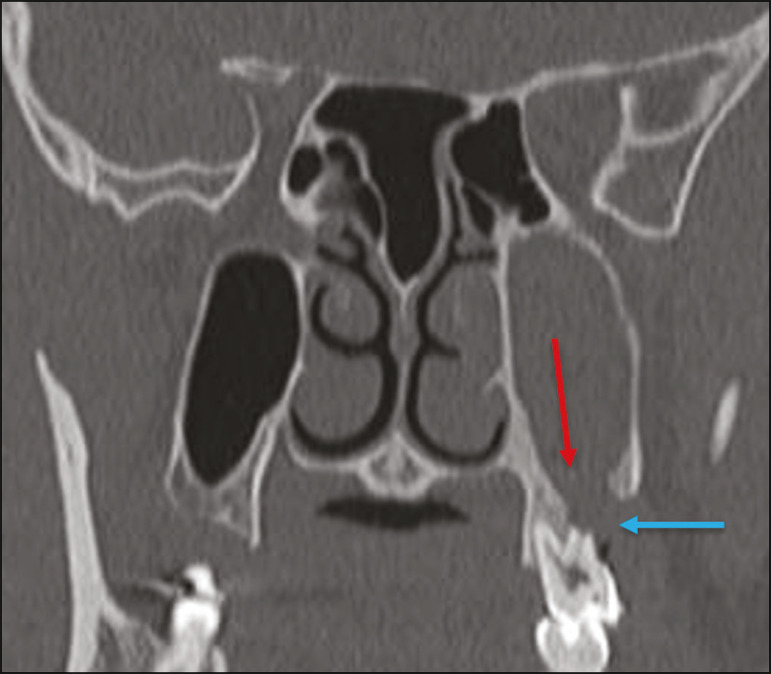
Coronal CT of the paranasal sinuses showing a bone defect in the floor of the left maxillary sinus (red arrow), which corresponds to an oroantral fistula causing odontogenic sinusitis. Note also the radicular amputation of the adjacent tooth (blue arrow).
